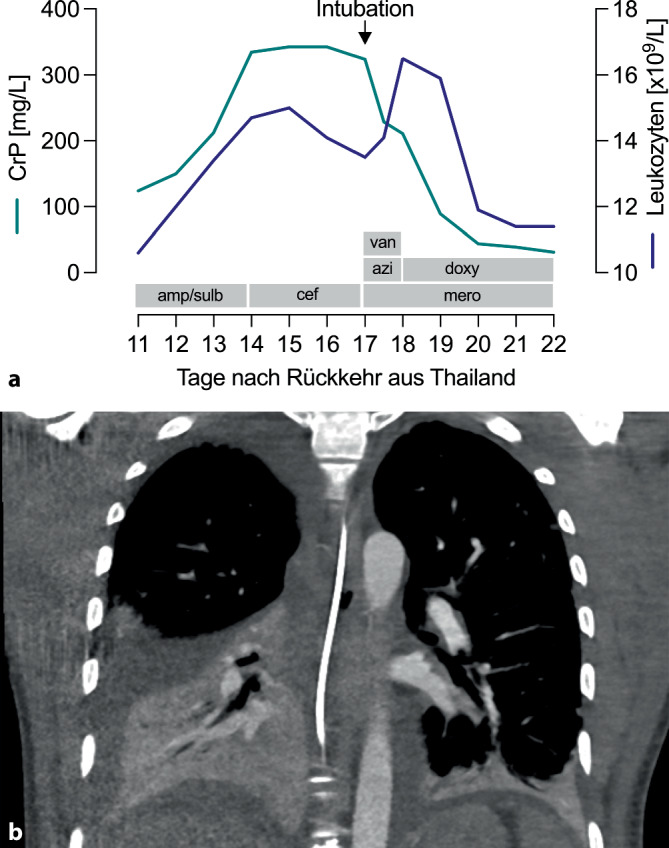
a Zeitverlauf von C‑reaktivem Protein (CrP) und Leukozytenzahl während der antiinfektiven Therapie mit Ampicillin/Sulbactam (*amp*/*sulb*), Ceftriaxon (*cef*), Meropenem (*mero*), Vancomycin (*van*), Azithromycin (*azi*) und schließlich Doxycyclin (*doxy*) nach positiver PCR für *Orientia tsutsugamushi*. b CT mit Konsolidierungen in beiden Unterlappen
